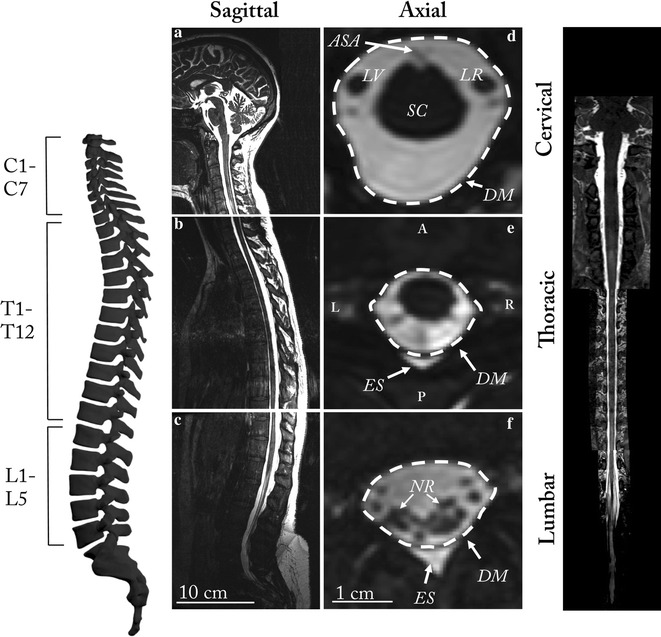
T2-weighted MRI data were collected as three volumes, a craniocervical, b thoracic, c Lumbosacral. A variety of artifacts exist in and around the SSS, d–f including the anterior spinal artery (ASA), left and right vertebral arteries (LV and LR), epidural space (ES), dura mater (DM), spinal cord (SC), and dorsal and ventral nerve rootlets (NR) in particular near the cauda equina. Note: the 3D geometry provided in this manuscript only includes the CSF within the spine below the foramen magnum (*L* left, *R* right, *A* anterior, *P* posterior)
